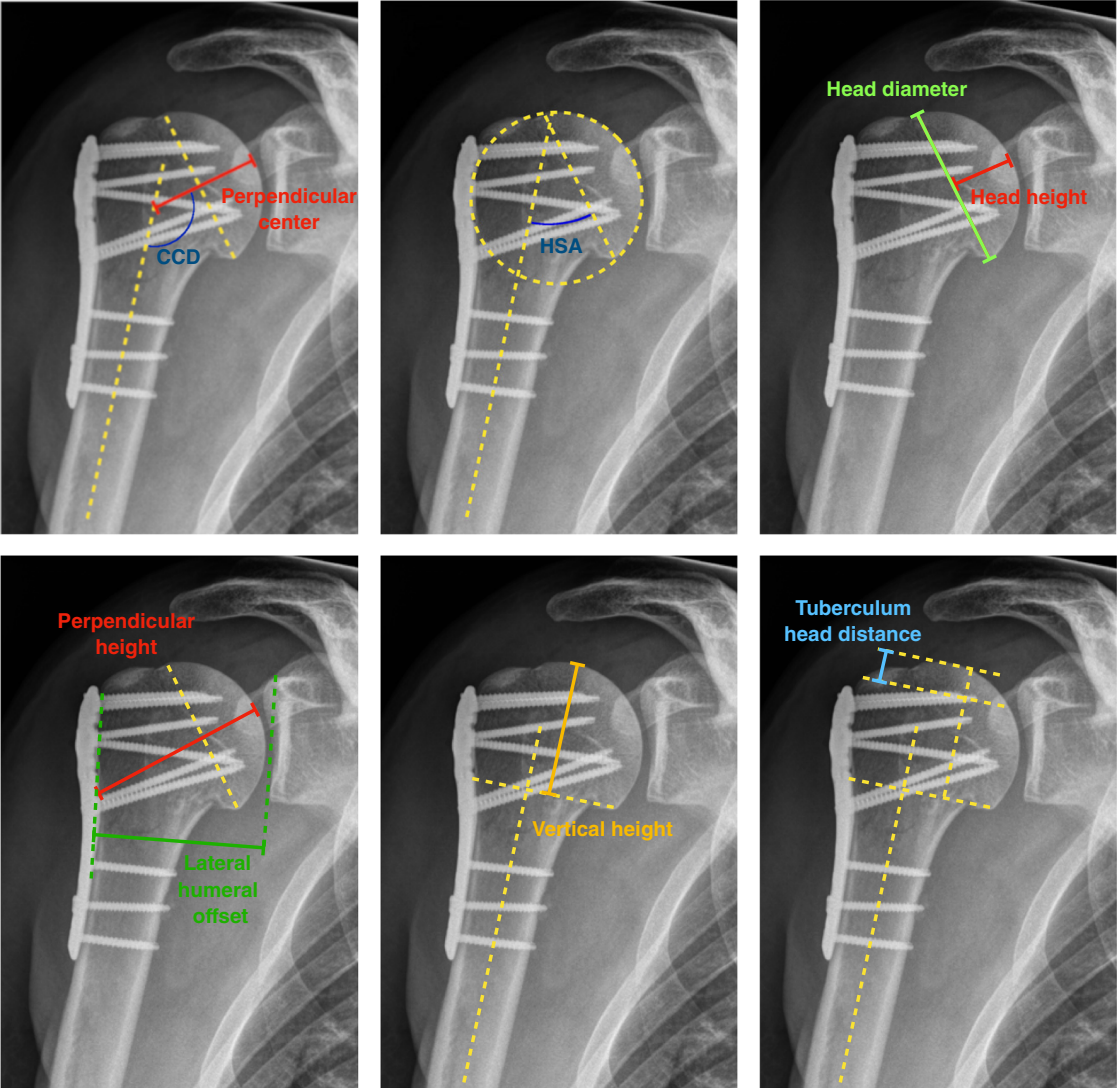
Overview of the measured radiographic parameters (dashed lines help to determine each parameter): Lateral humeral offset, distance between tuberculum and head apex, head diameter, head height, perpendicular height, perpendicular center, vertical height, and angles between head and humeral shaft (CCD and HSA)
